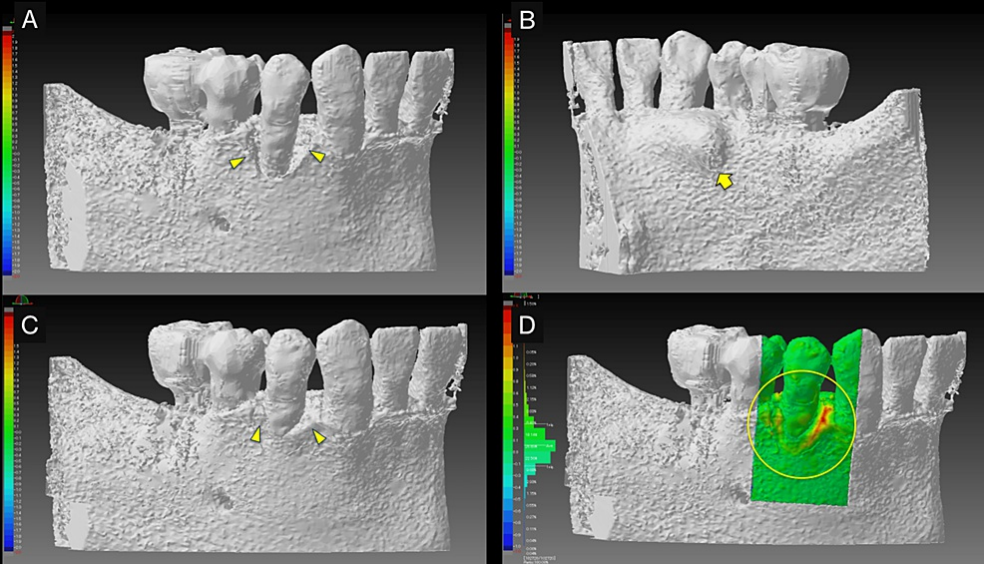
3D views of pre- and post-operative cone-beam computed tomography (CBCT) images and superimposed images of pre- and post-operative CBCT images. (A) Preoperative CBCT images show intrabony defects (arrowheads). (B) A mandibular torus on the lingual side of tooth #44, would have made it difficult to assess the intrabony defect on the intraoral radiographic image (arrow). (C) CBCT image at 14 months postoperatively shows a bone-like structure in the intrabony defect on the buccal side of tooth #44. (D) In the preoperative-postoperative superimposition, areas suggestive of bone regeneration are shown in yellow to red (circle).
